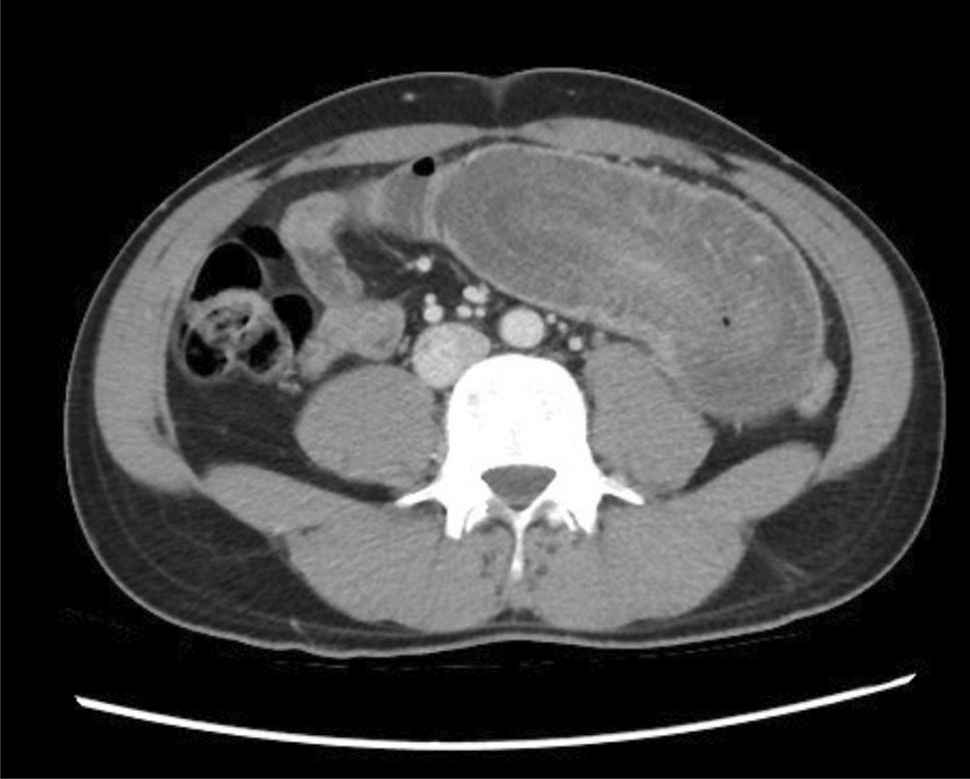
CT scan abdomen with contrast showed a long segment entero-enteric (jejuno-jejunal) intussusception seen mainly in the left side of the abdomen
